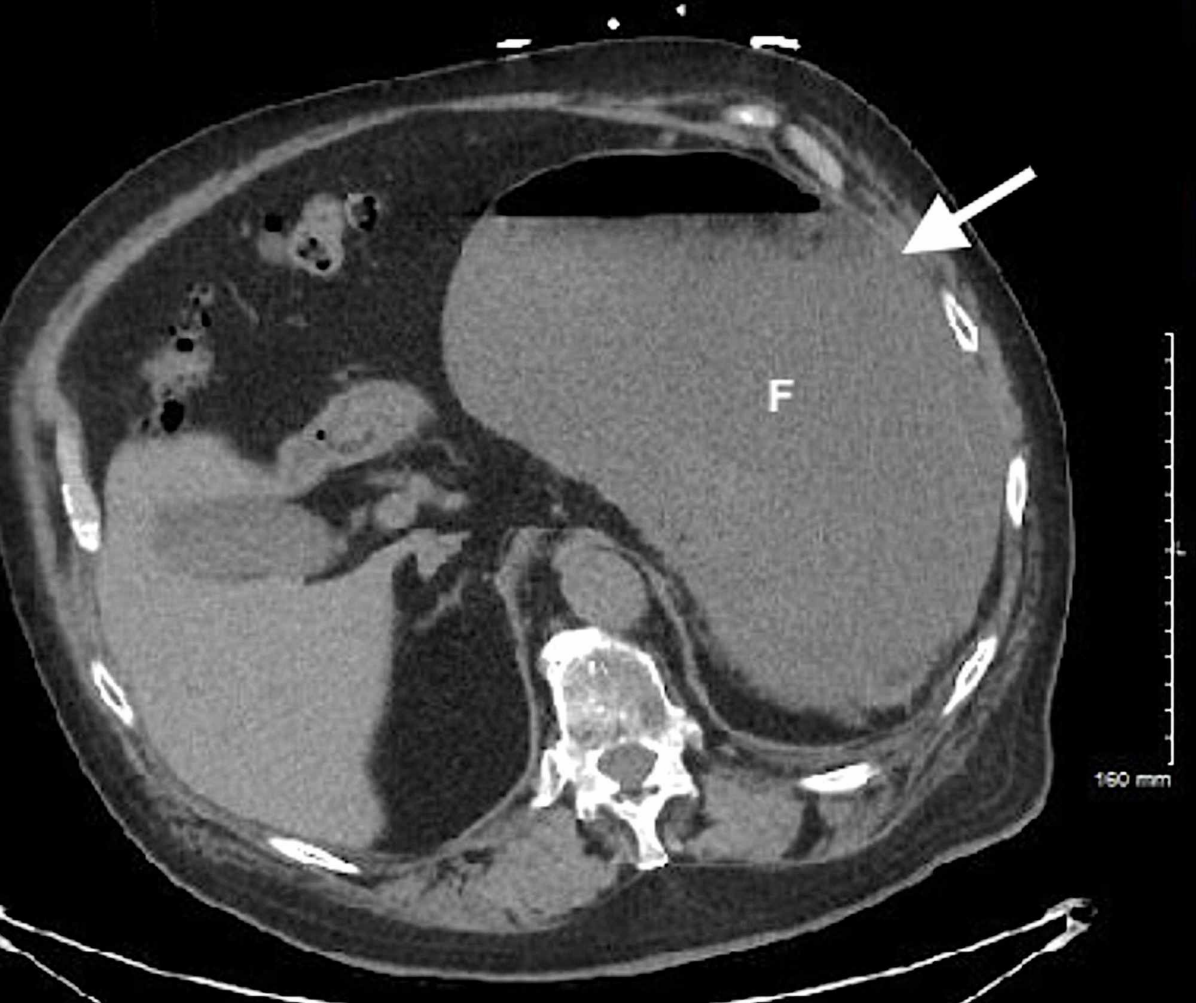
CT of the abdomen/pelvis (axial view) demonstrating gastric volvulus with a fluid-filled distended stomach (solid arrow). F, gastric fundus.
